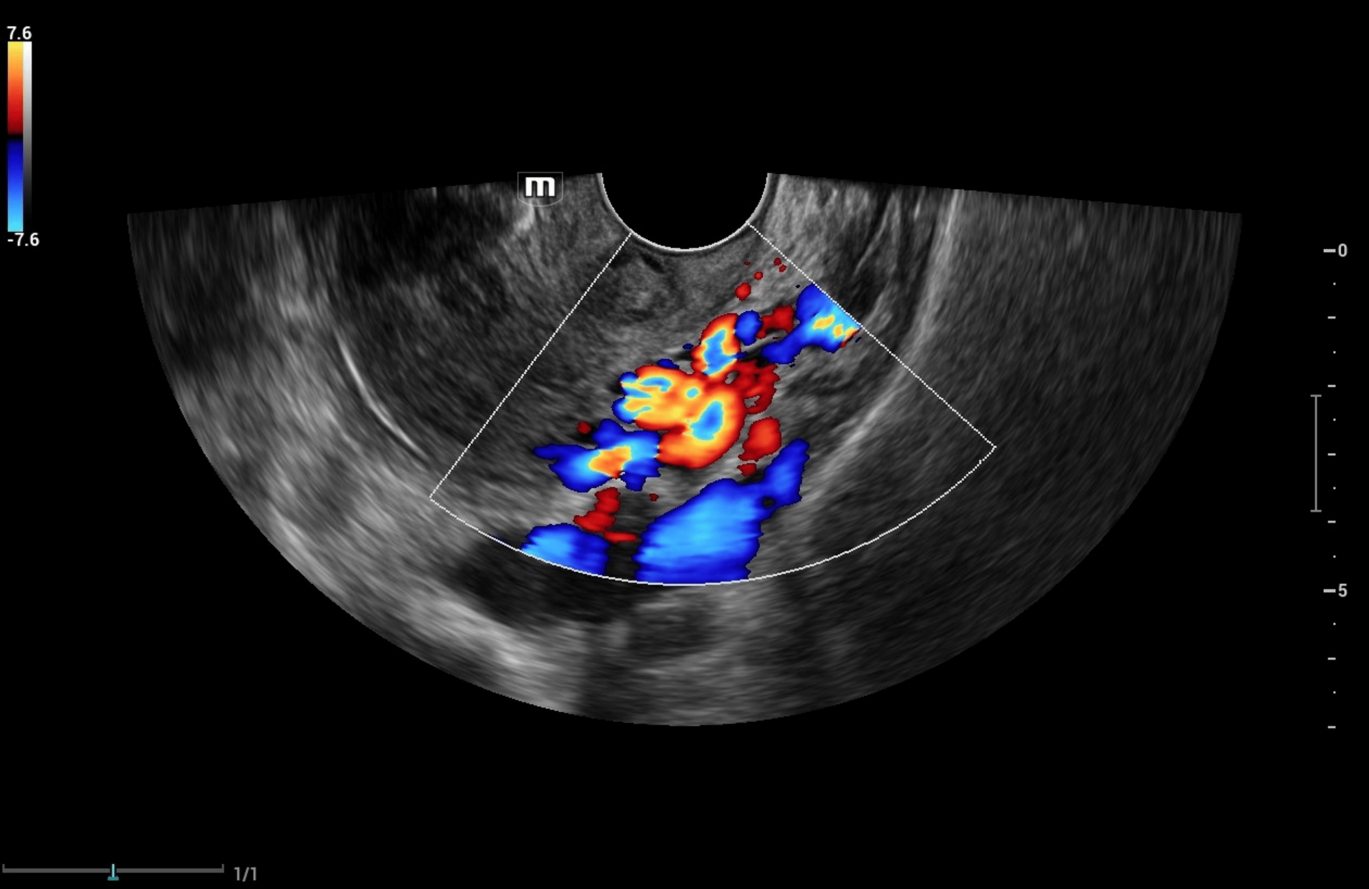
Color Doppler ultrasound shows dilated veins in the adnexae.
